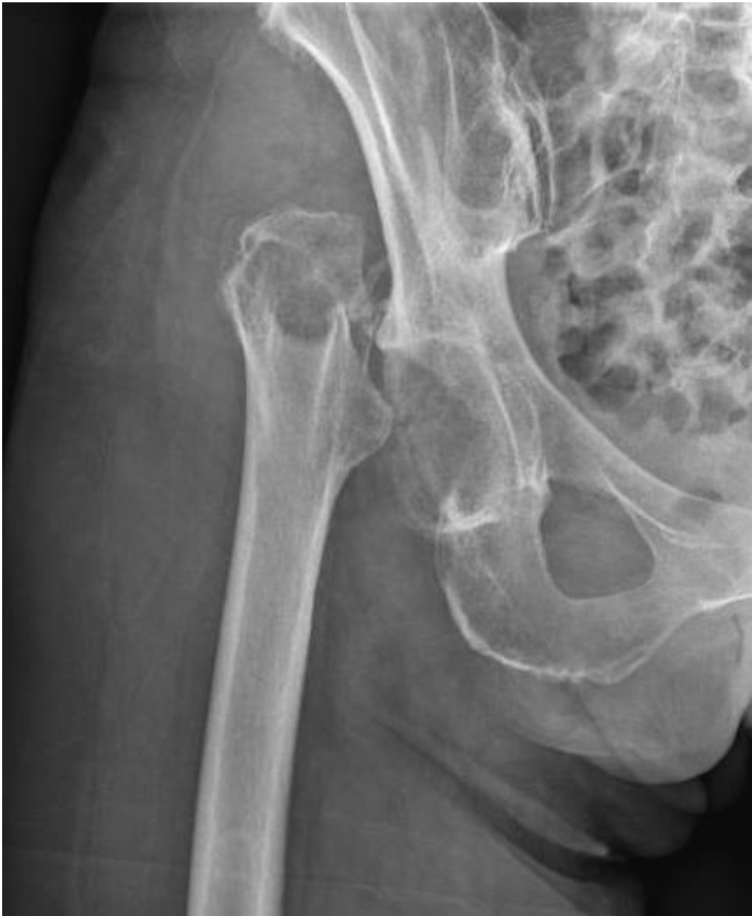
Plain X-ray after removal of the total hip arthroplasty. A prosthesis-free interval of 6 weeks was performed.
